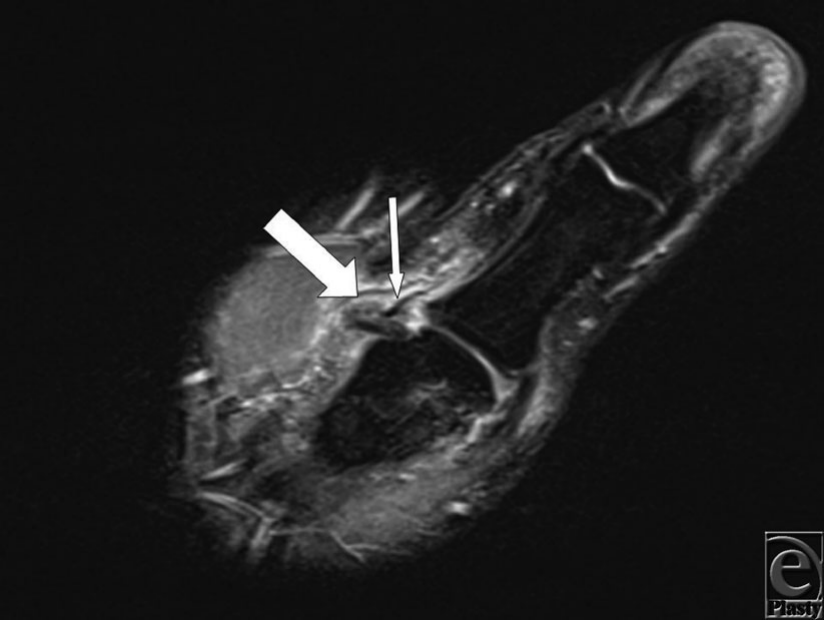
Coronal T2 weighted fat-suppressed MRI of the thumb demonstrating the ulnar collateral ligament (thick white arrow) subluxed ulnar to the adductor aponeurosis (thin white arrow).
